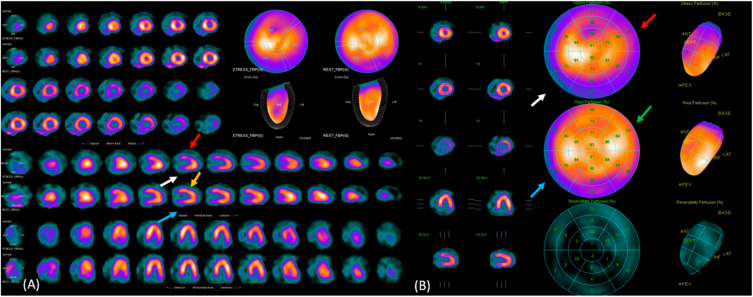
Myocardial perfusion SPECT demonstrating viable myocardium in LAD and RCA territories. (A) Stress images (short-axis, vertical long-axis, and horizontal long-axis views) showing partial perfusion defects in the anterior wall (red arrow) and obvious ischemia in the inferior wall (white arrow). Rest images demonstrated reversible ischemia in the anterior wall (yellow arrow) and partial reversible ischemia in the inferior territory (blue arrow). (B) Corresponding stress, rest, and reversibility polar maps demonstrated inducible ischemia in both vascular distributions, especially in the LAD territory (red and green arrows) and moderate reversible perfusion in the RCA territory (white and blue arrows), consistent with viable myocardium.
